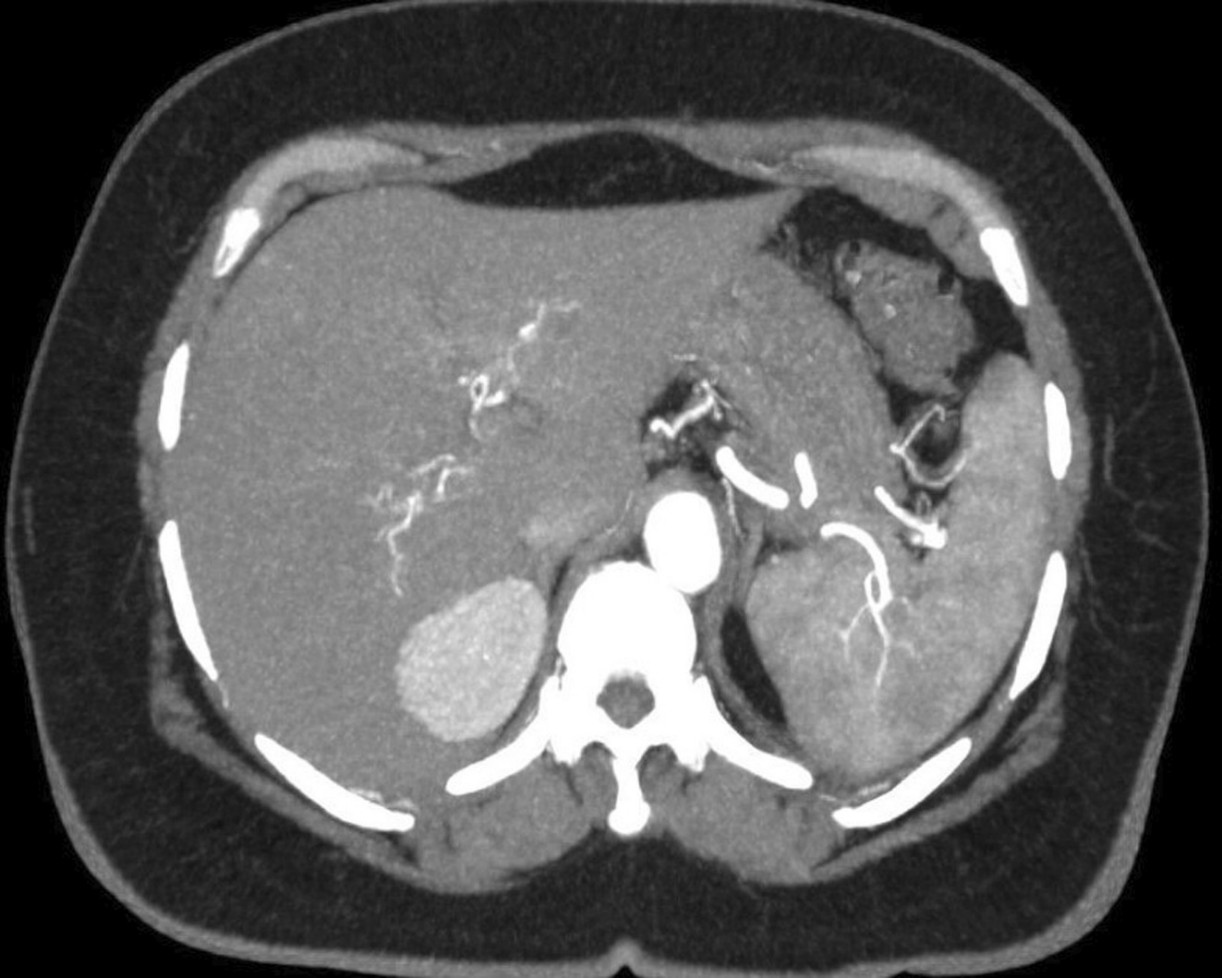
In the final image of the contrast‐enhanced aortic CT angiography, all aneurysms had spontaneously regressed and no vascular pathology was observed.
